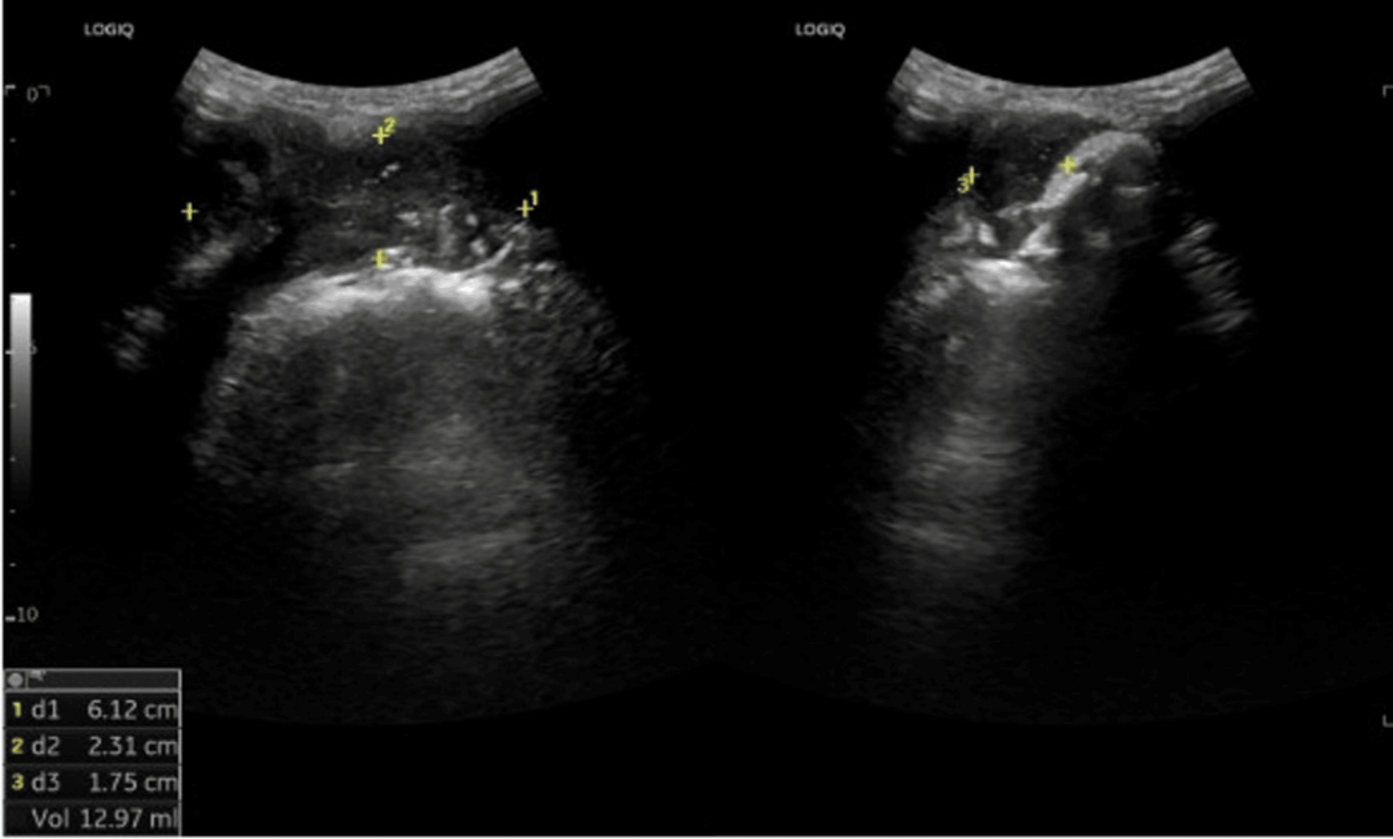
Ultrasound of the right submandibular region showing a heteroechoic collection (6.1 × 2.3 × 1.7 cm; ~13 mL) with internal echoes and posterior enhancement, suggestive of abscess.
